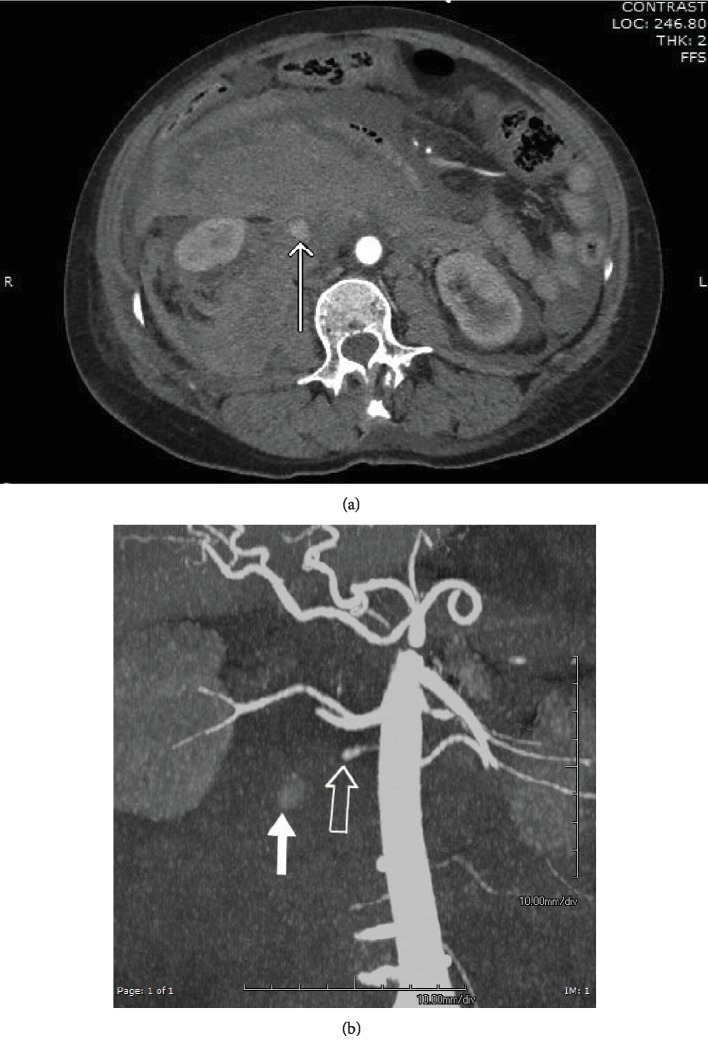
(a) Axial image from contrast-enhanced CT demonstrates collection of contrast suggestive of pseudoaneurysm (arrow) with surrounding massive retroperitoneal hemorrhage. (b) Coronal maximum intensity projection image from CT scan shows partial filling of vessel arising from the aorta presumed to be the right ovarian artery (open arrow) and collection of contrast suggestive of pseudoaneurysm (solid arrow).
